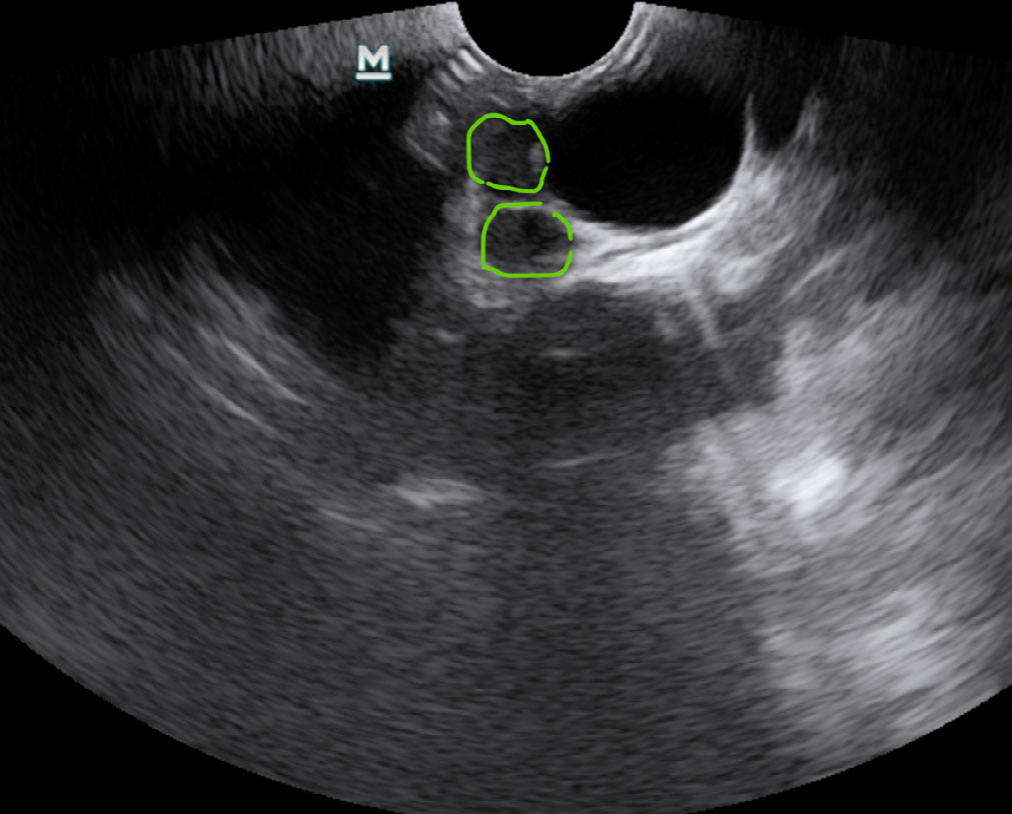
Two pathological lymph nodes ultrasonographically.
